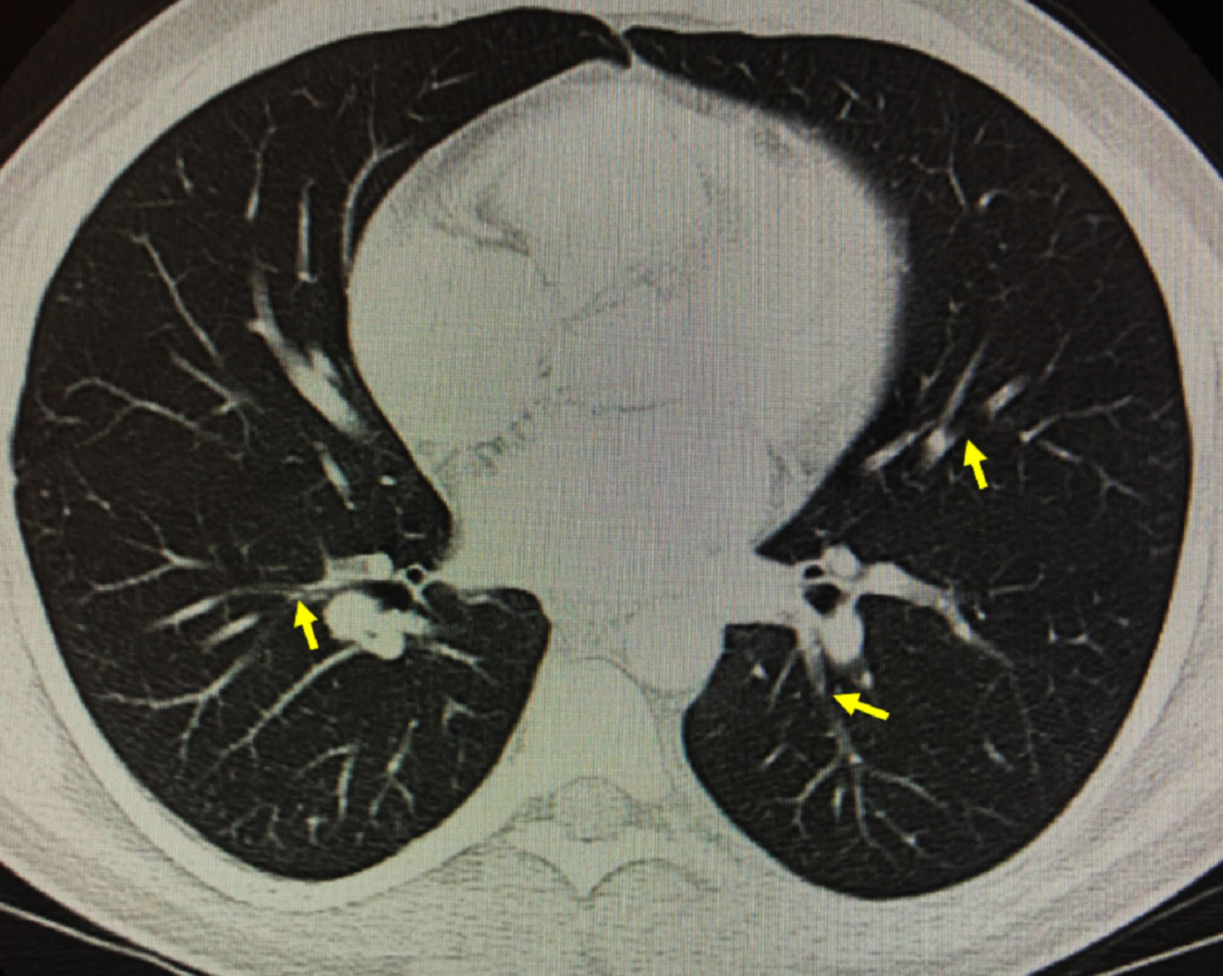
Computed tomography angiography of the chest Cross-sectional representative image of the chest. Notable subtle small bilateral filling defects within the pulmonary branches are indicated with the yellow arrows.
